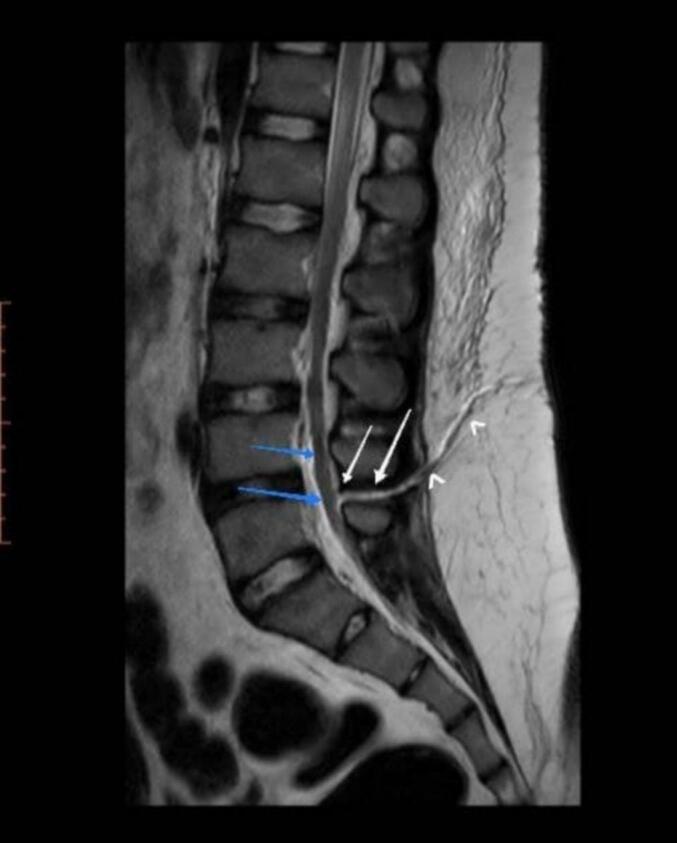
Axial CT scan of the lower lumbar region, white arrows show penetrating trauma at interspinous L4-L5 space, and the fistula tract extends from subcutaneous up to epidural space of spinal canal at L4-L5 level. Blue arrows show nerve roots clumping. (For interpretation of the references to colour in this figure legend, the reader is referred to the web version of this article.)
